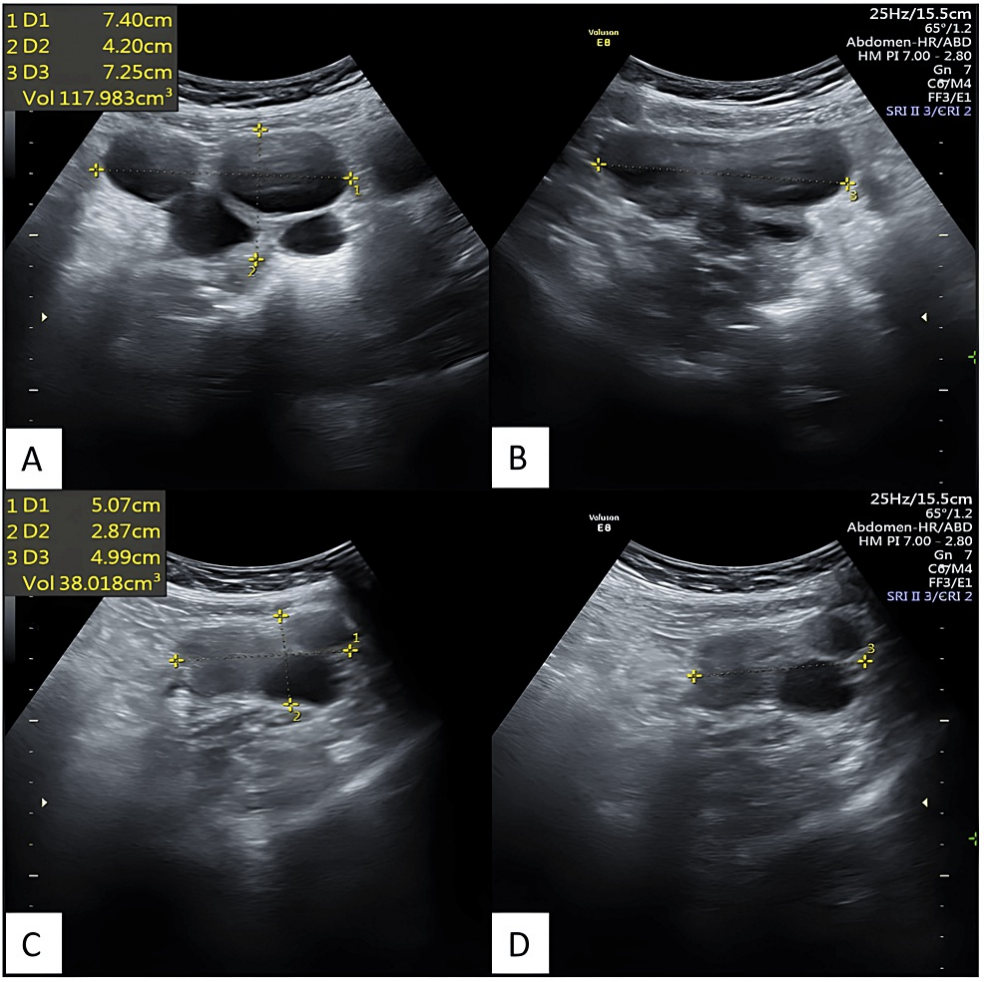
Transabdominal ultrasonography images depict bilateral ovaries with multiple cysts, taken after 34 days of thyroid replacement therapy. The volumes of the ovaries have been reduced compared to the previous scan. Transabdominal ultrasonography reveals an enlarged right ovary in axial (A) and sagittal (B) sections, as well as an enlarged left ovary in axial (C) and sagittal (D) sections, both exhibiting multiple cysts. The volumes of the ovaries have been reduced compared to the previous scan.
